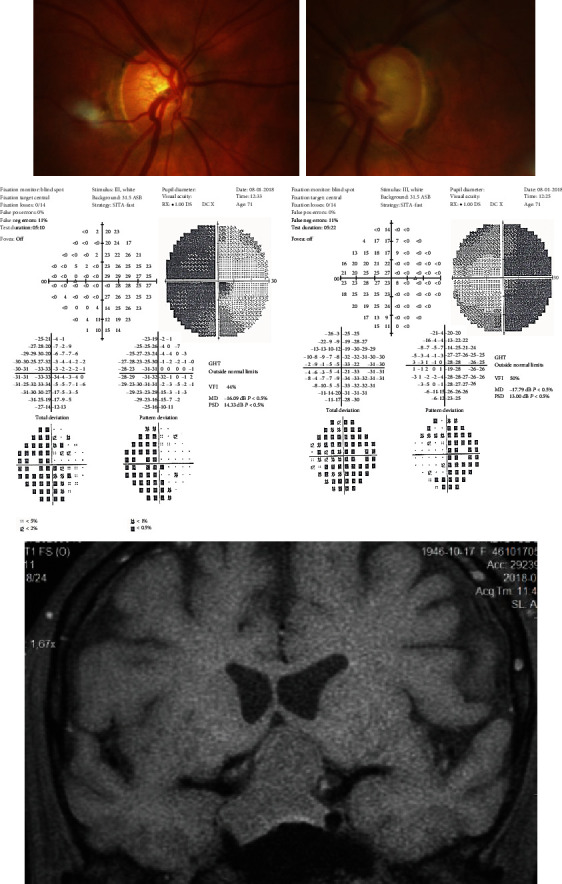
F/68 referred with glaucoma progression, neuroimaged because of bitemporal hemianopia. MRI scans revealed pituitary adenoma compressing and elevating optical chiasm.
